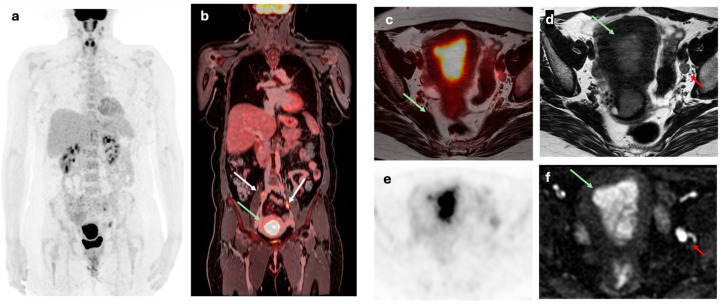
A 50-year-old woman with a FIGO IIIC1 EC. (a) Maximum intensity projection (MIP) and (b) coronal fused [^18^F]F-FDG PET/MRI staging images showing primary endometrial tumor with bilateral common iliac lymph node involvement (white arrows) and left external iliac node metastasis. (c) Axial fused [^18^F]F-FDG PET/MRI, (d) axial T2-weighted, (e) axial [^18^F]F-FDG PET, and (f) DWI images showing a heterogeneous on T2 with diffusion restriction of primary tumor (green arrow) and a 9 mm left external iliac lymph node with pathological FDG uptake (red arrow).
